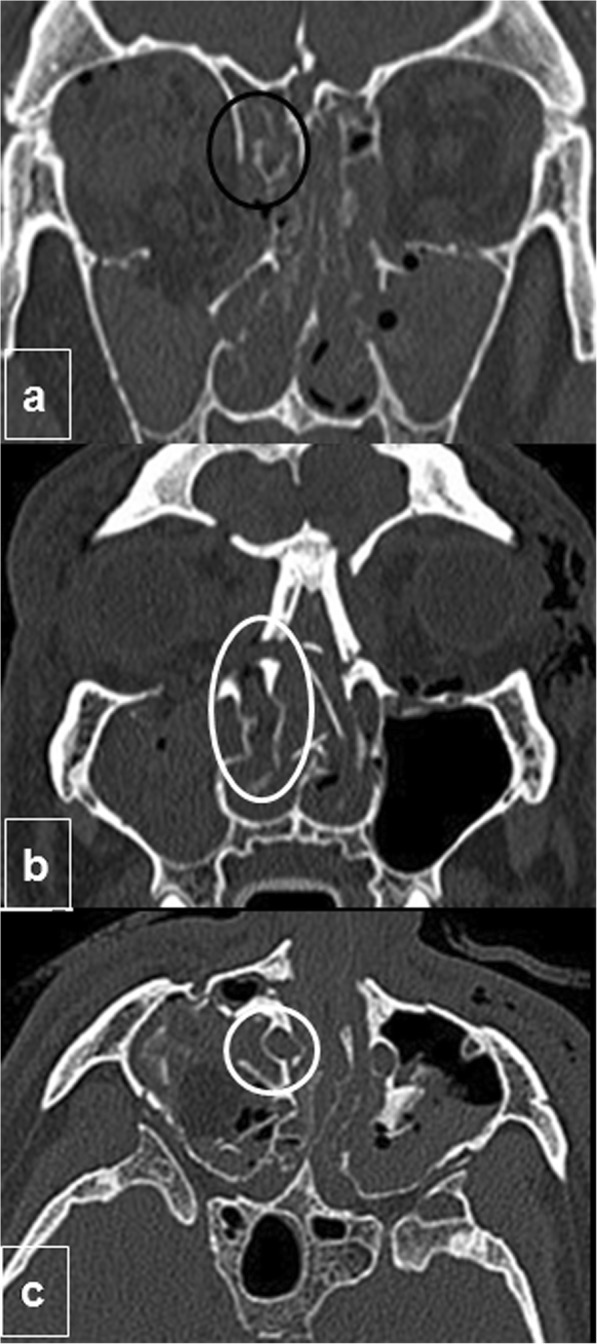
Complications involving drainage canals. a Coronal CT image shows frontal recess involvement by fracture (black circle). b Coronal and (c) axial CT images at the level of the maxillary sinus showing extension of naso-orbito-ethmoidal complex fractures through both nasolacrimal ducts on the right side (circled in white)
